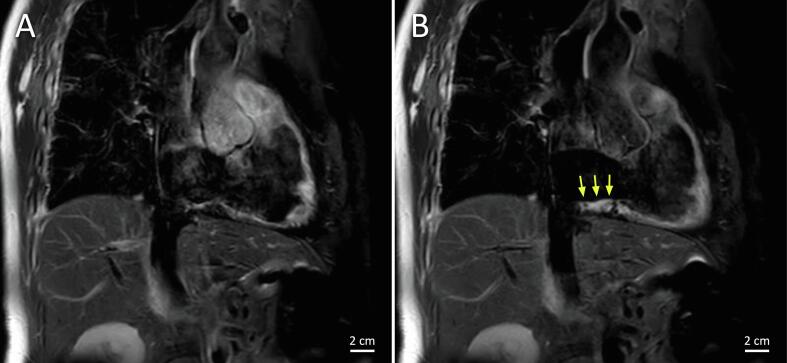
T2-weighted MR images of the CTI in the RAO view before
